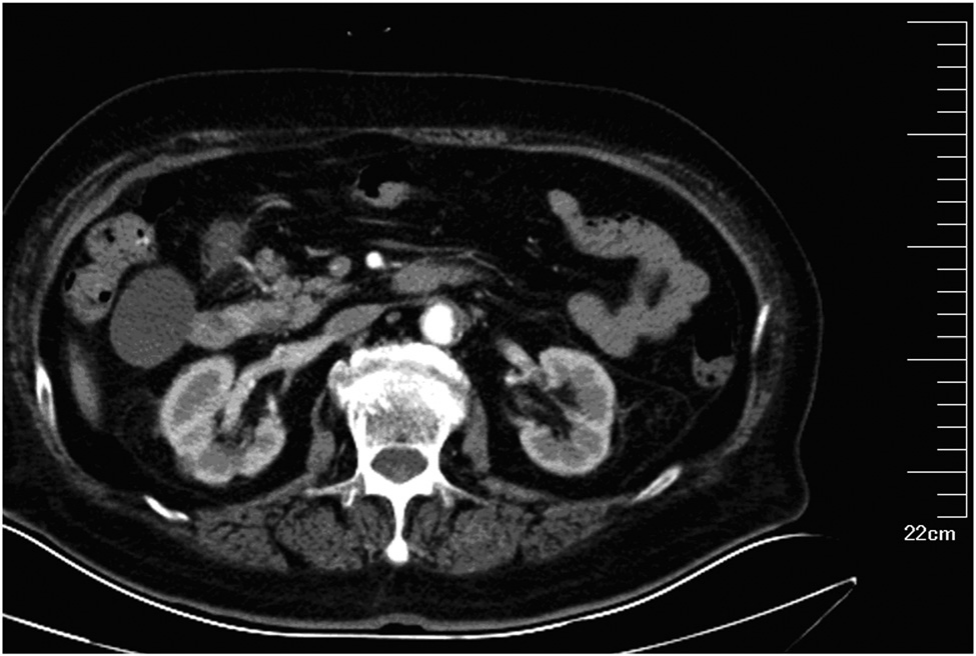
An abdominal CT scan showed kidney cysts and a little exudation around the kidneys.
